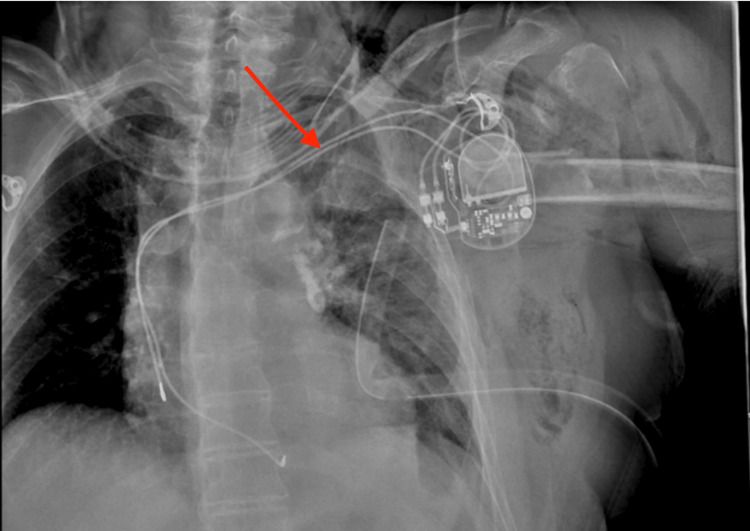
X-ray showing the intrathoracic humeral head fracture (red arrow)
